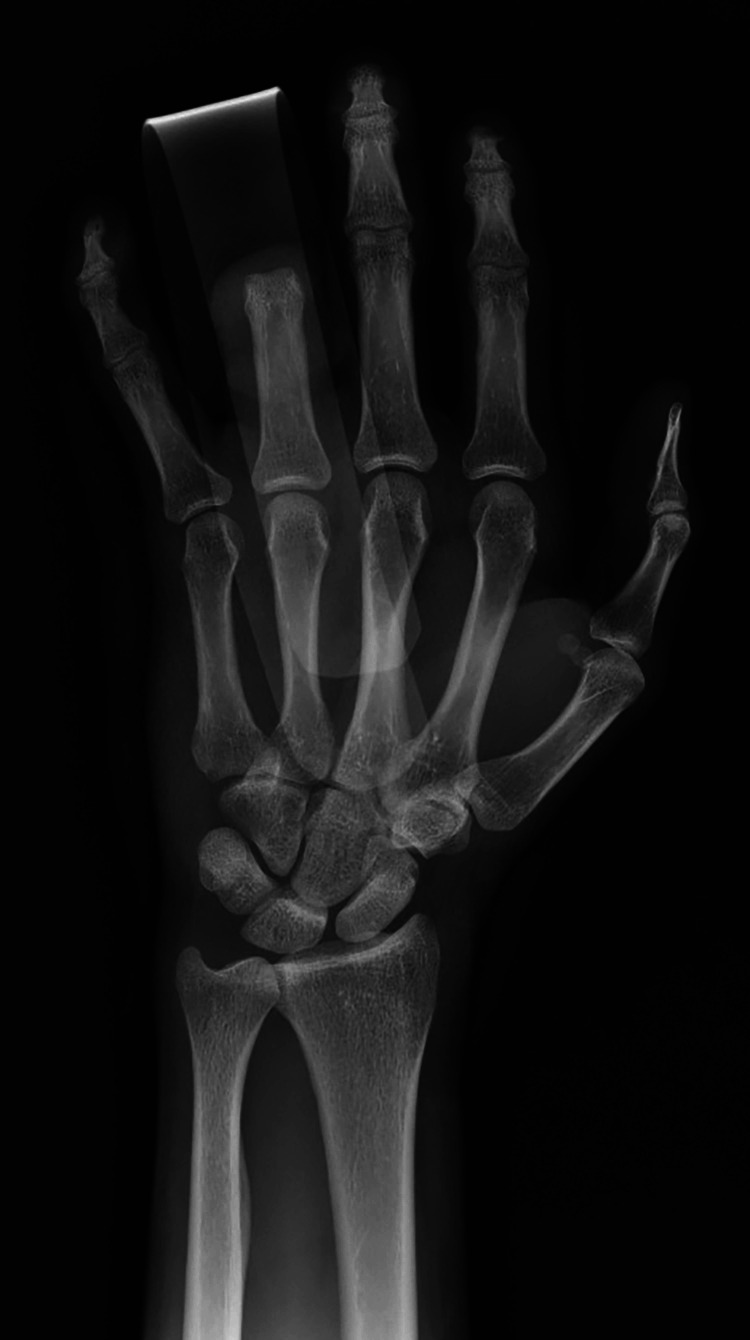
Posteroanterior plain radiograph of the left hand demonstrating revised amputation to the level of the proximal interphalangeal joint post-splint.
